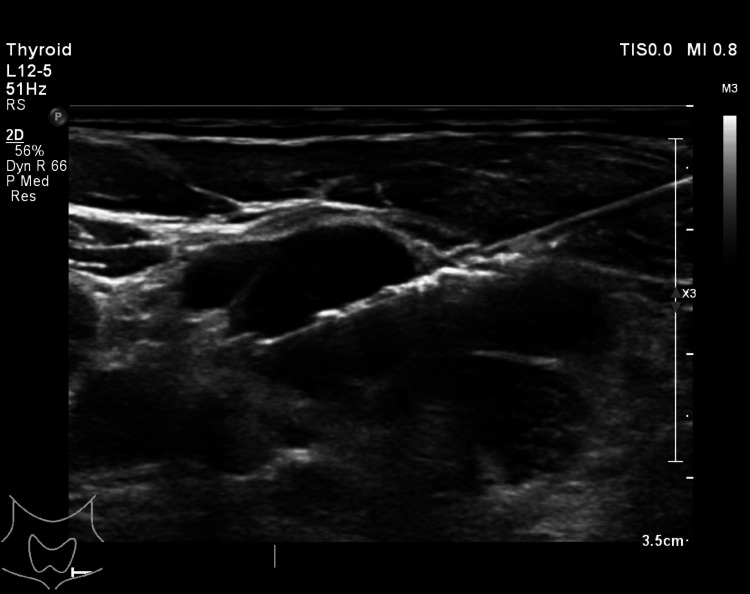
Ultrasound showing enlarged left cervical lymph node
